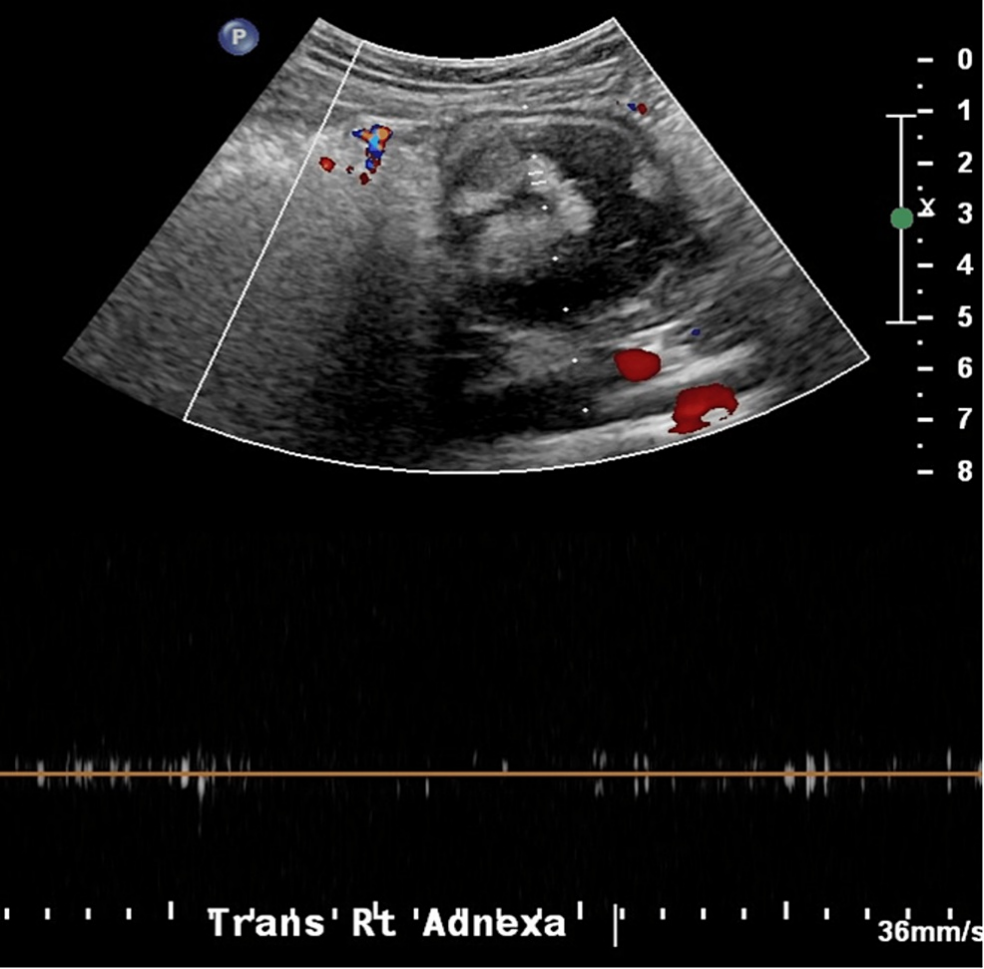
Doppler ultrasound of the right ovary showing a 5-cm teratoma but with normal waveform and color Doppler flow
